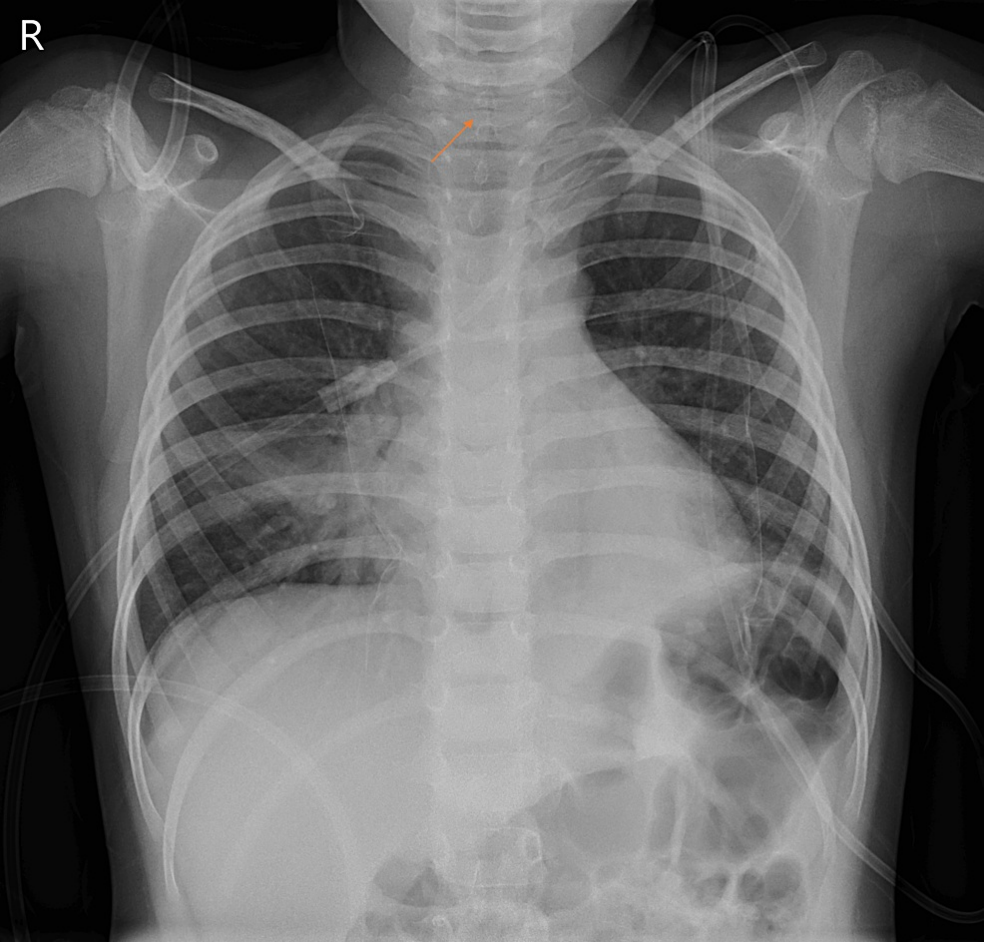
Anteroposterior x-ray showing narrowing of subglottic airways, known as steeple sign
